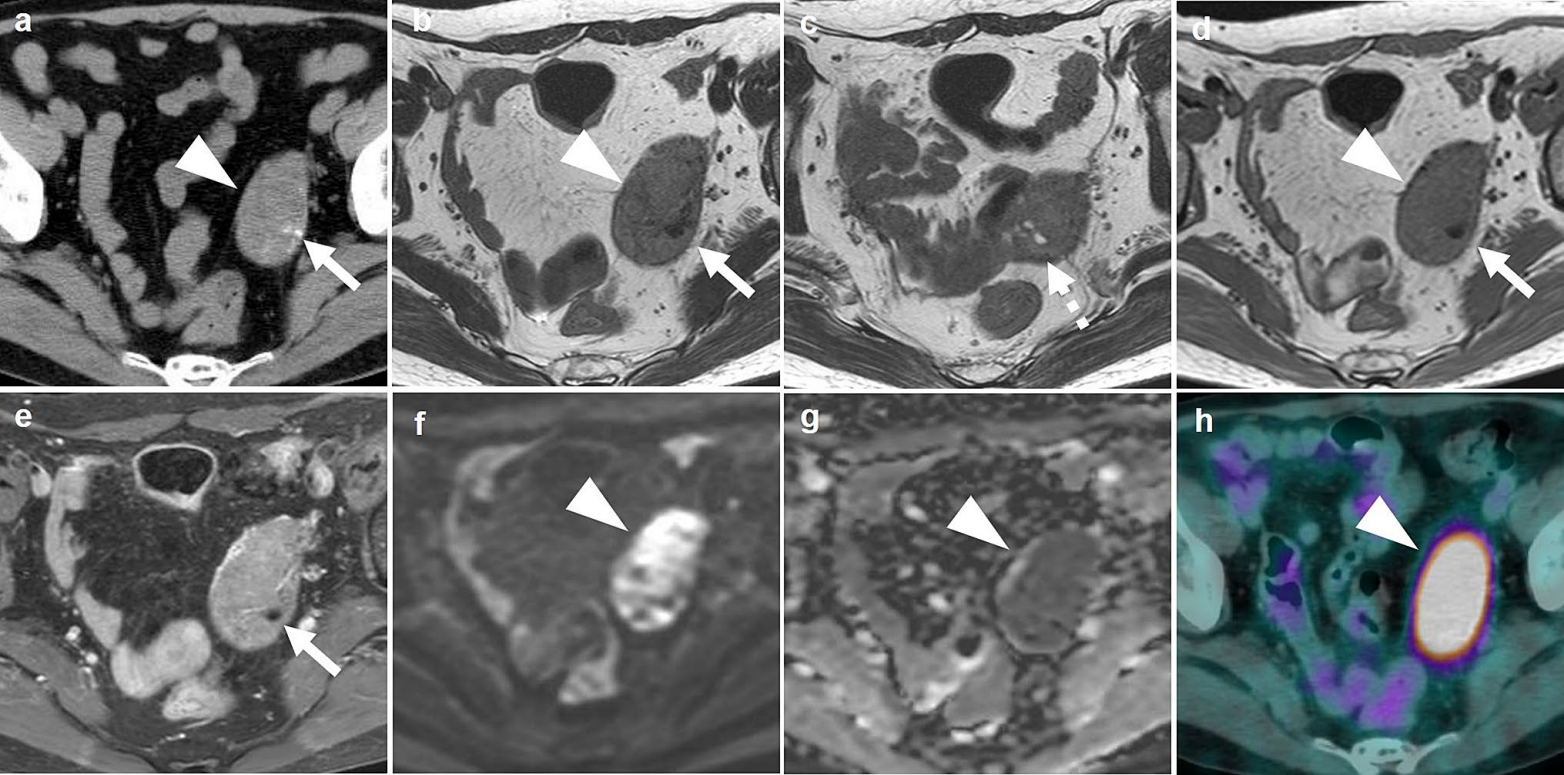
A 66-year-old asymptomatic woman who has diabetes mellitus. a Axial unenhanced CT shows a well-defined tumor (arrowhead) of predominantly soft-tissue attenuation, containing small foci of calcification (arrow). b Axial T2 weighted MR image demonstrates an entirely solid tumor (arrowhead) of intermediate intensities along the left pelvic wall, containing an area of hypointense dot corresponding to the calcification on CT (arrow). c Another axial T2-weighted image at caudal level shows cystic components of hyperintensities (dashed arrow). d T1-weighted image shows the tumor of iso-intensities (arrowhead) compared with an area of hypointense dot corresponding to the calcification (arrow). e Fat saturated contrast-enhanced T1-weighted image shows homogeneous enhancement in the solid portions except for the focus of calcification (arrow). f Diffusion-weighted image at b-value of 1000 shows heterogeneous high intensities within the tumor (arrowhead). g ADC map image shows decreased apparent diffusion coefficient (ADC) value (0.57 × 10^–3^ mm^2^/s) in the tumor (arrowhead). h The fused PET/CT image shows homogeneous FDG accumulation, measuring SUV max of 8.2 (arrowhead).
